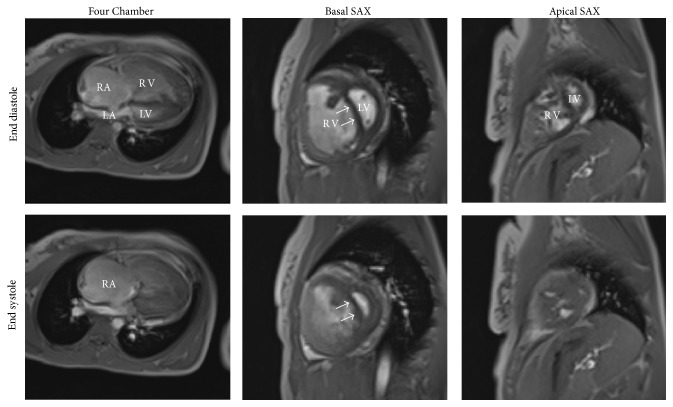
MRI images at end diastole and end systole. The images show markedly enlarged right ventricle (RV) and right article (RA) with leftward interventricular septal wall bowing (arrows). The RV is significantly trabeculated, especially at the apex. LV = left ventricle; LA = left atrium; SAX = short-axis.
